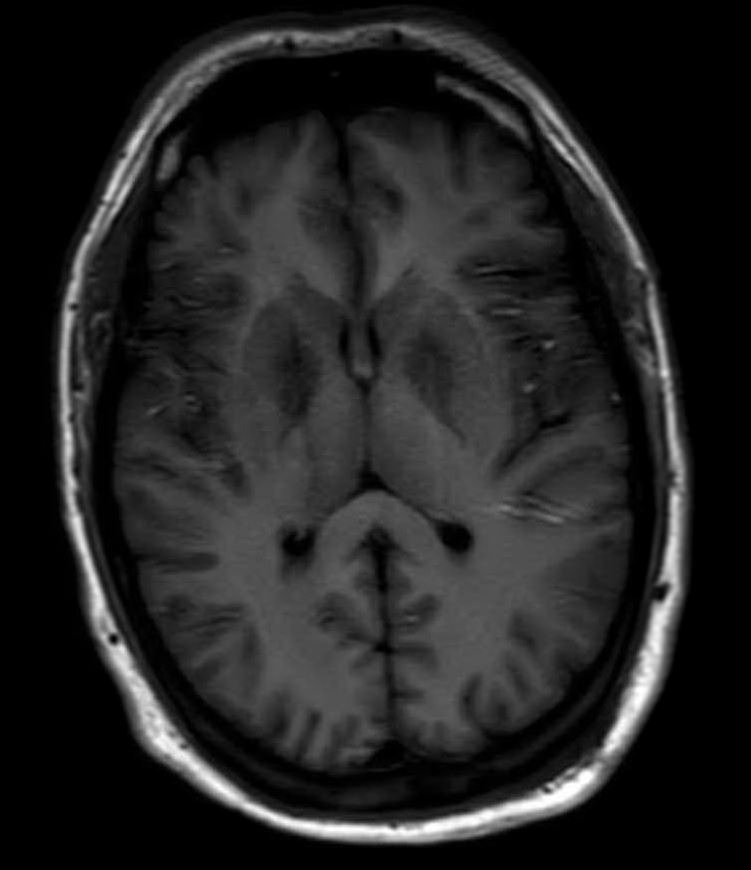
T1 FLAIR MRI Brain Showing Hypointense GP Relative to Surrounding Brain Parenchyma
